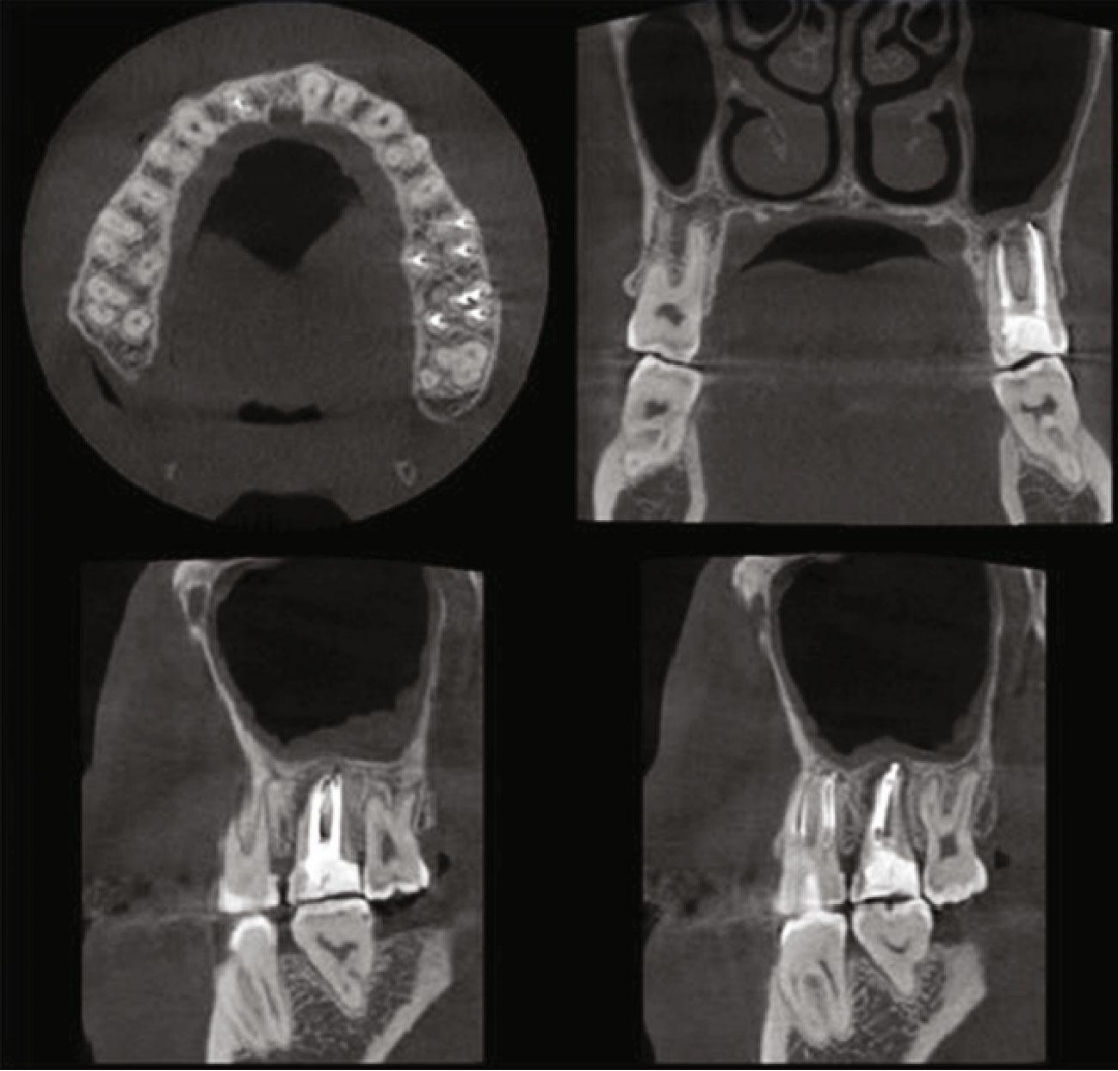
CBCT after obturation. Axial, coronal, and sagittal views.
